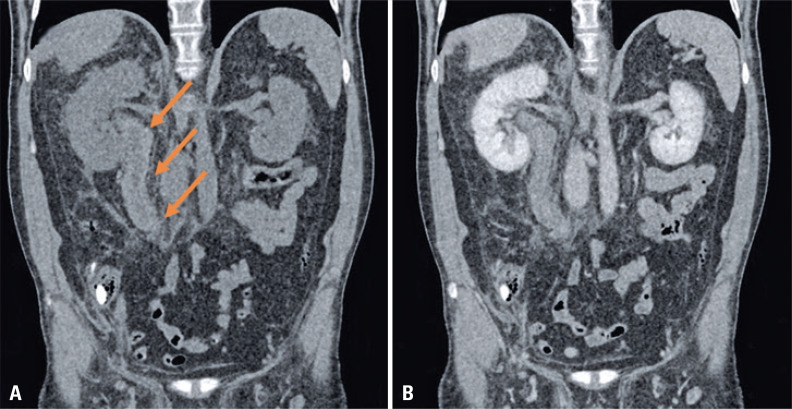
Abdominal computed tomography in coronal view. (A) unenhanced image shows a hyperattenuating circumferential thickening of the right ureter wall in practically its entire extension with adjacent fat-stranding; (B) contrast-enhanced image shows no enhancement of the ureteral wall. These findings are compatible with an intramural hematoma. It should be highlighted that given the hyperattenuation of the subepithelial hematoma, it should be mistaken by a diffuse enhancement of the ureteral wall if the non-contrast phase is not performed
